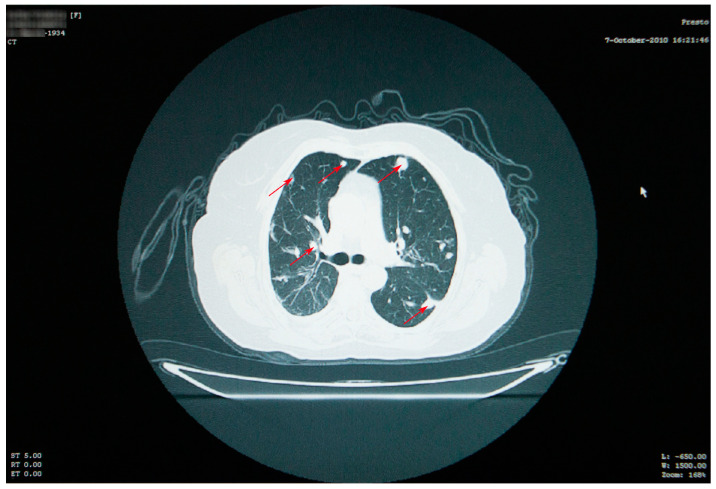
Thoracic CT showing a reduction in the appearance and dimensions of the cysts (red arrows), which is associated with favorable evolution under treatment.
